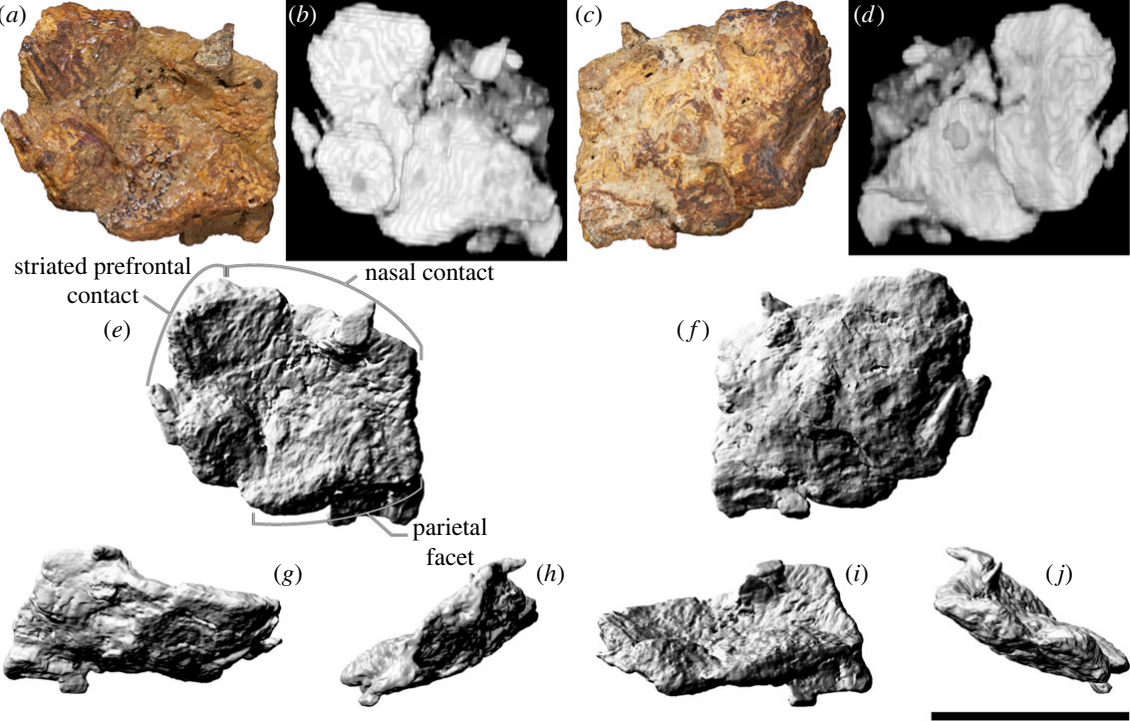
Diamantinasaurus matildae* referred left frontal (AODF 0906). (*a–d*) Left frontal in (*a*,*b*) dorsal and (*c*,*d*) ventral views. (*a*) and (*c*) are photographs, (*b*) and (*d*) are three-dimensional models derived from CT data. (*e–j*) Three-dimensional models (derived from surface scans) of the left frontal in (*e*) dorsal, (*f*) ventral, (*g*) anterior, (*h*) medial, (*i*) posterior and (*j*) lateral views. Scale bar = 50 mm.
